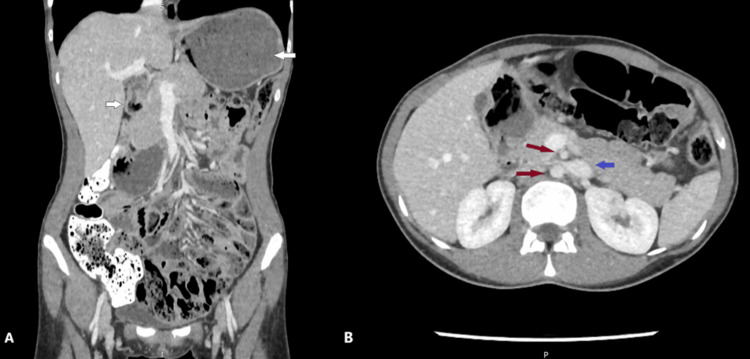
Computed tomography scan (A) Coronal plane. The findings confirm gastric and duodenal dilation, as well as the presence of air-fluid levels proximal to the duodenal stenosis (white arrows). (B) Axial plane. The upper and lower red arrows point to the superior mesenteric artery and abdominal aorta, respectively, and the blue arrow indicates the left renal vein
